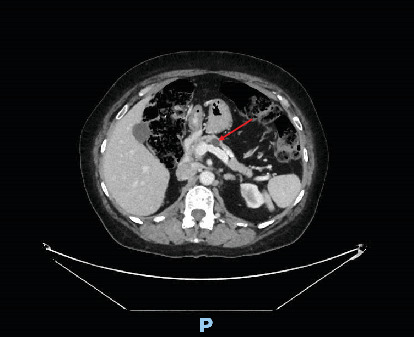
Contrast-enhanced CT of the abdomen and pelvis showing a 1.2 cm cystic lesion within the body of the pancreas.
